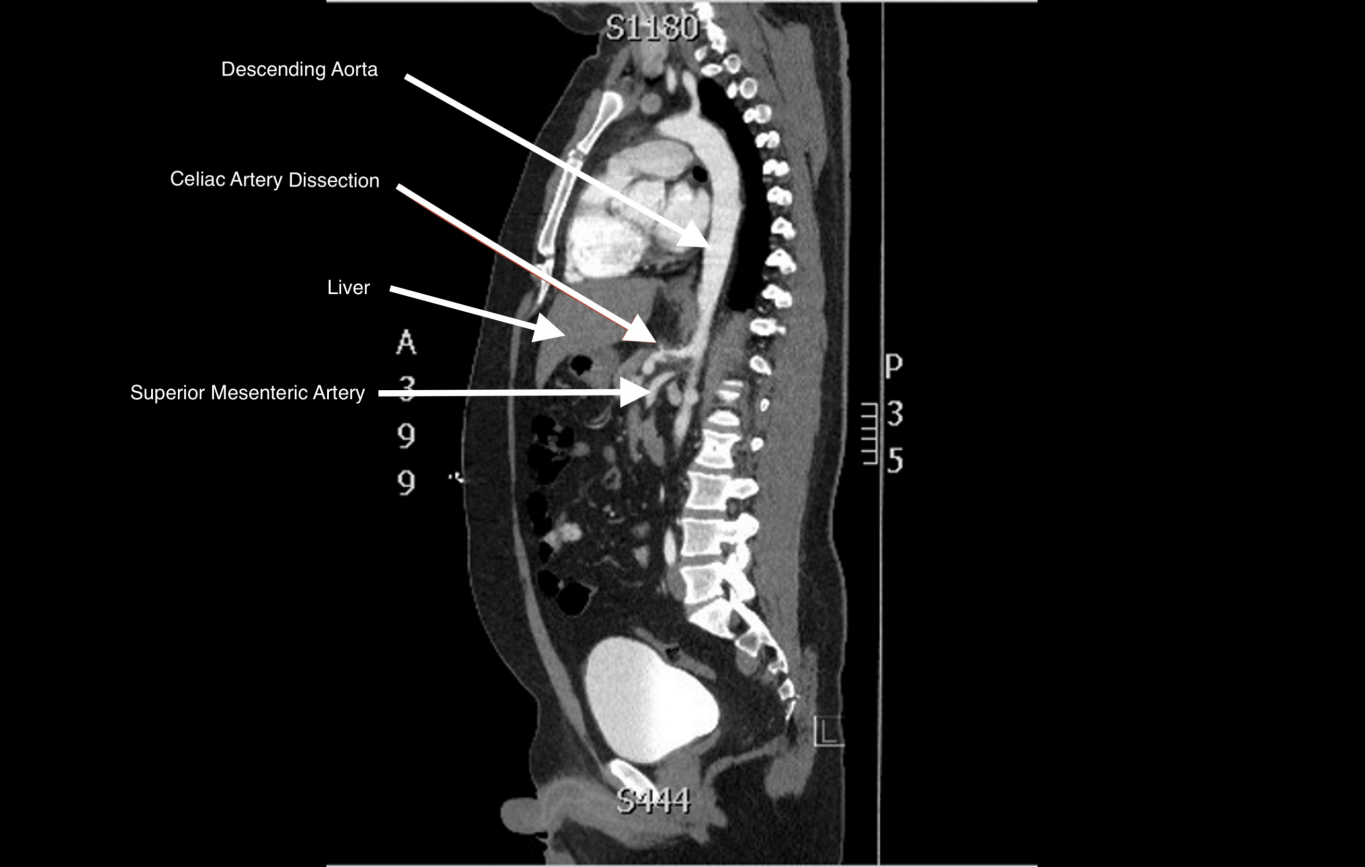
Computed tomography scan in the candy cane view
